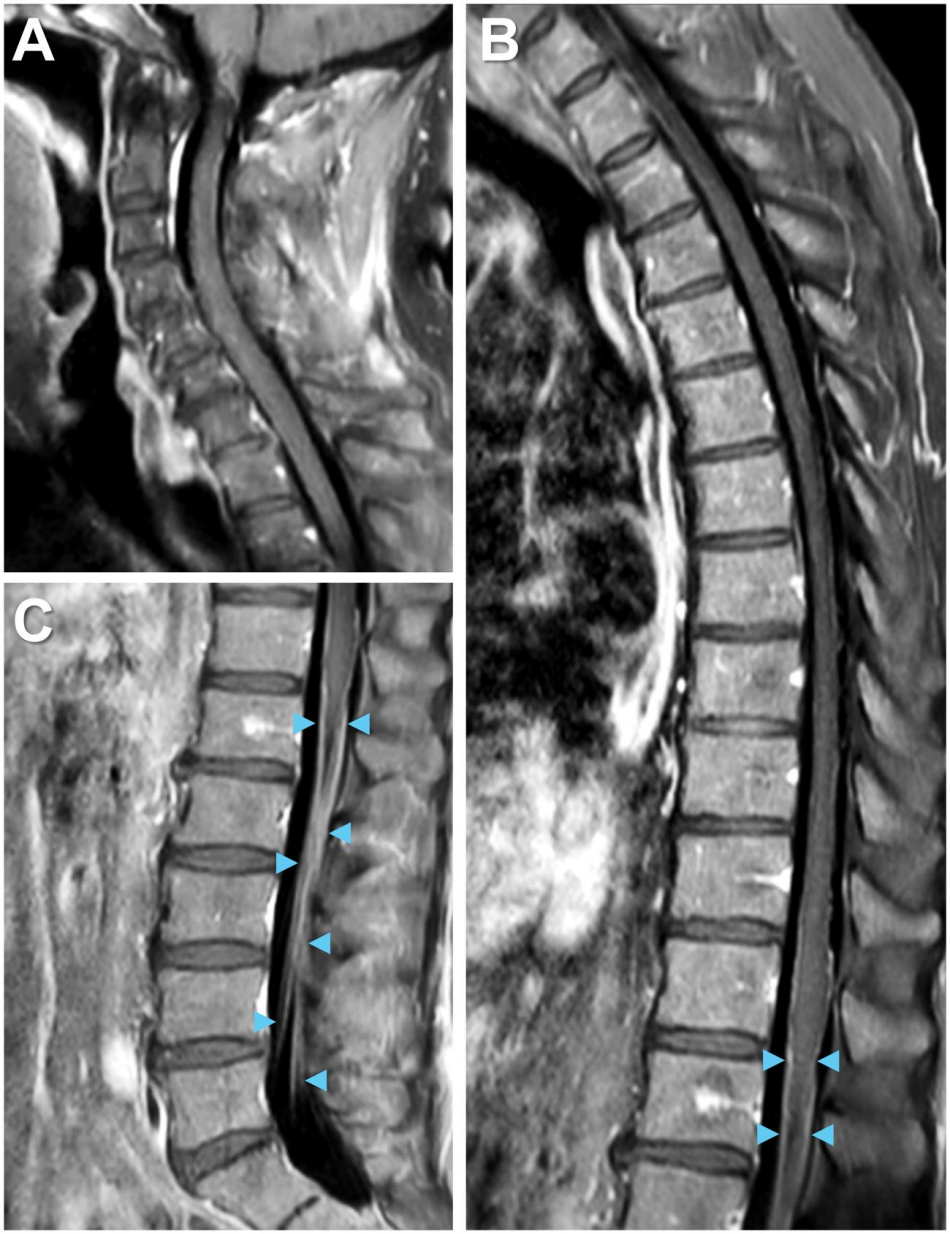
Complete spine imaging on MRI. The majority of contrast enhancement is not readily seen in the (A) cervical or (B) thoracic but rather the (C) lumbar region on T1 post-contrast spine imaging. Enhancement of bilateral cauda equina nerve roots (arrowheads).
